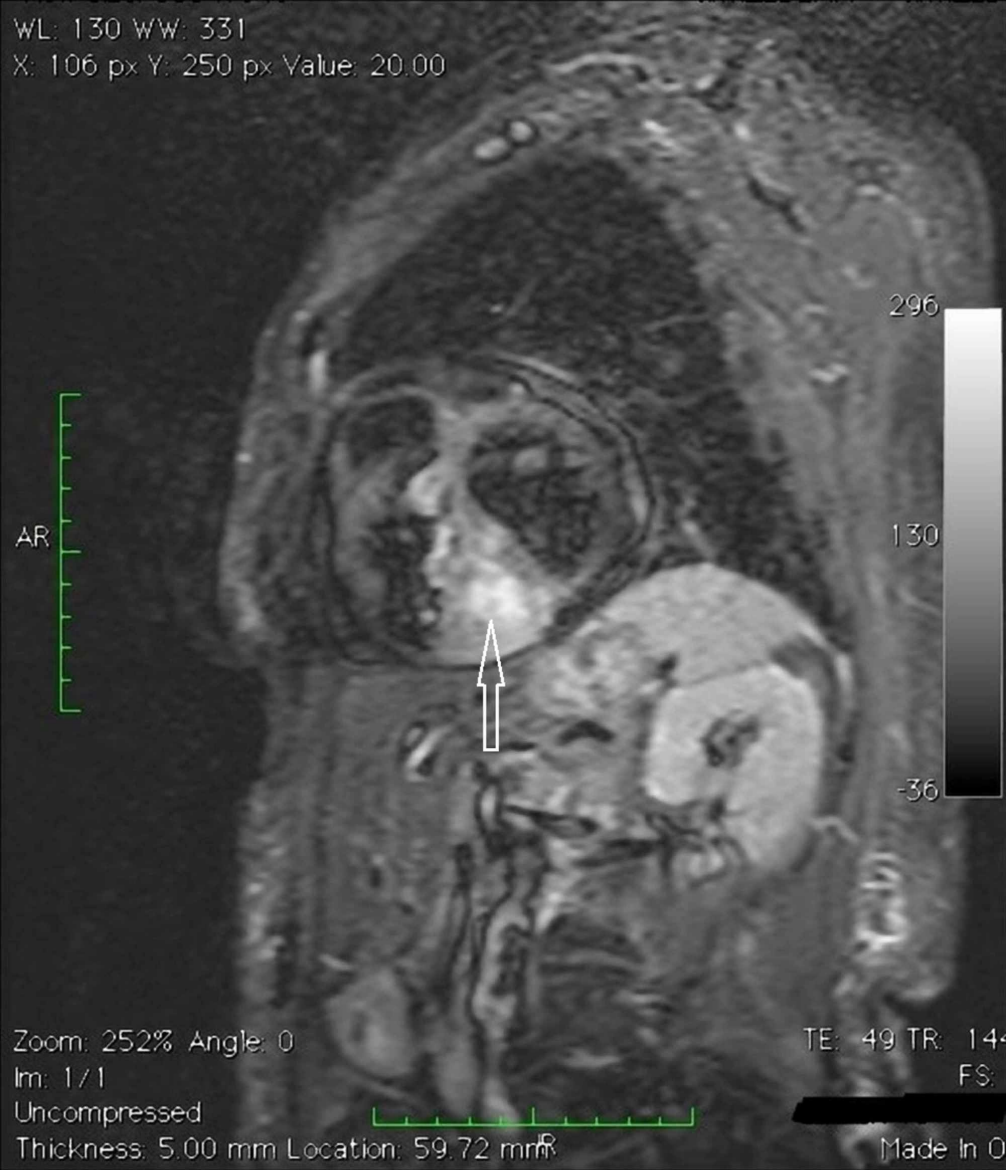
Cardiac magnetic resonance imaging (MRI) Turbo inversion recovery magnitude (TIRM) MRI. Confirmation of the expansive formation already observed on computed tomography-scan, another expansive lesion in the free wall of the right ventricle; evidence of necrosis within the mass.
